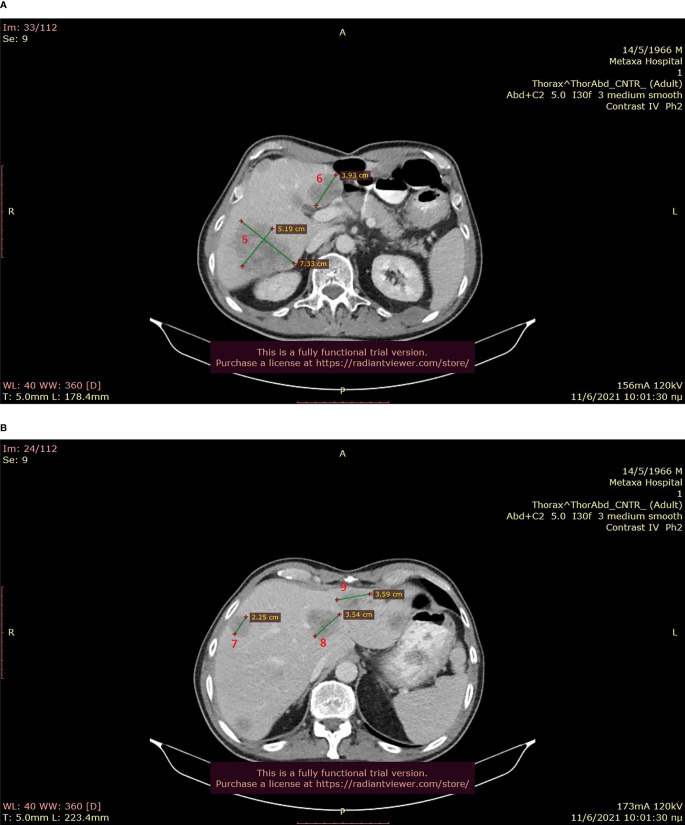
(A, B): Baseline abdominal CT showing multiple hepatic metastases (June 2021).
